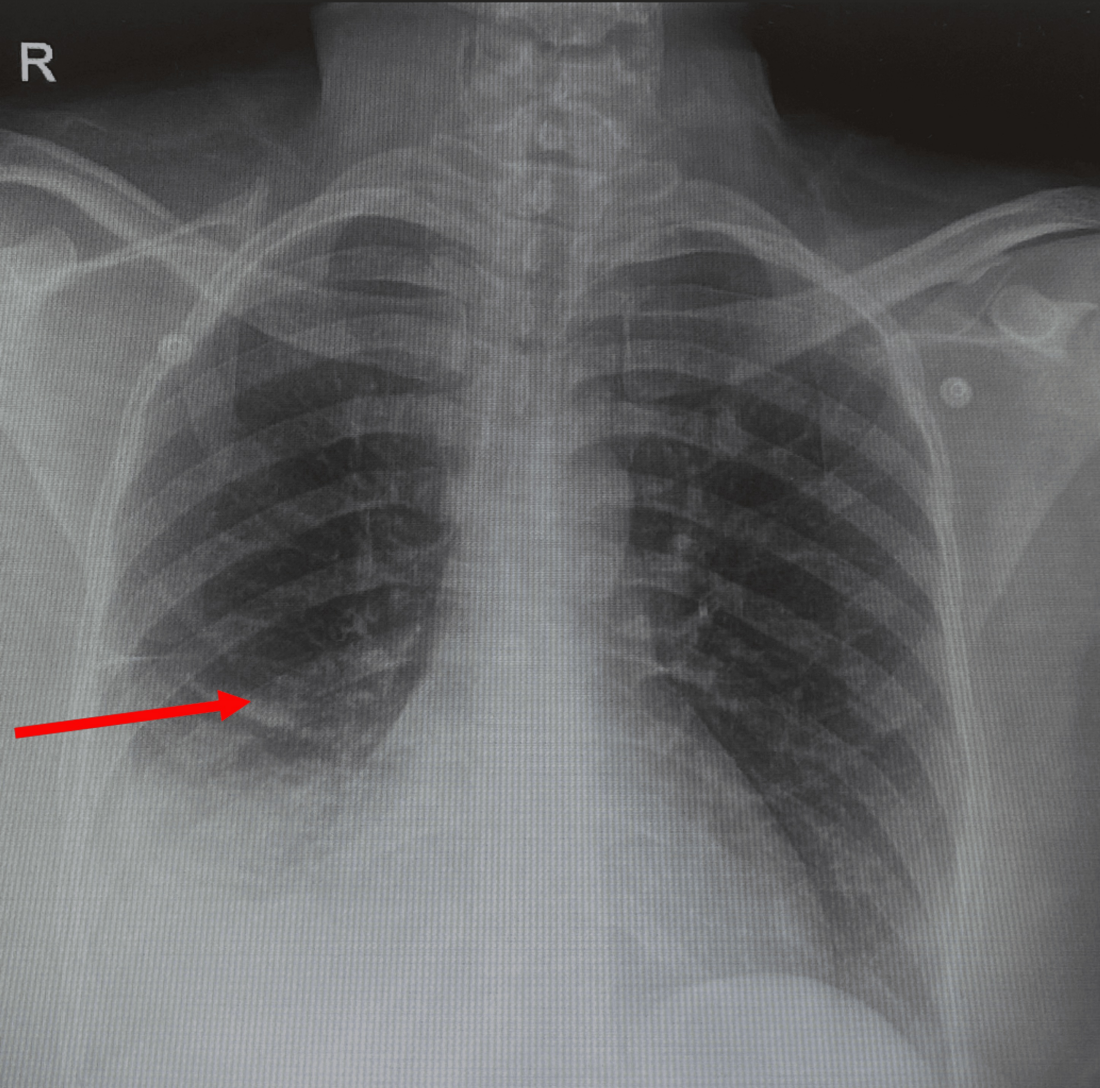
Chest Radiograph Showing Resolution of Recurrent Large Right-Sided Pleural Effusion After Chest Tube Insertion Red arrow highlighting the area of interest.
